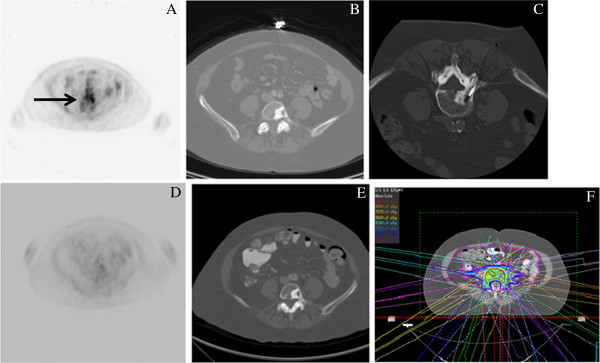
PET response in a lesion non-measurable by CT. Pretreatment PET (A) and CT (B) of a patient with a bony metastatic lesion. The black arrow points to the area of PET avidity on the pretreatment PET (A). PET (D) and CT (E) 1 month after completion of SBRT shows what seems to be stable disease (SD) on CT, but RECIST criteria cannot be used for bony lesions. Post treatment PET shows almost complete response (CR). Pretreatment biopsy (C) and SBRT plan (F) are also depicted.
